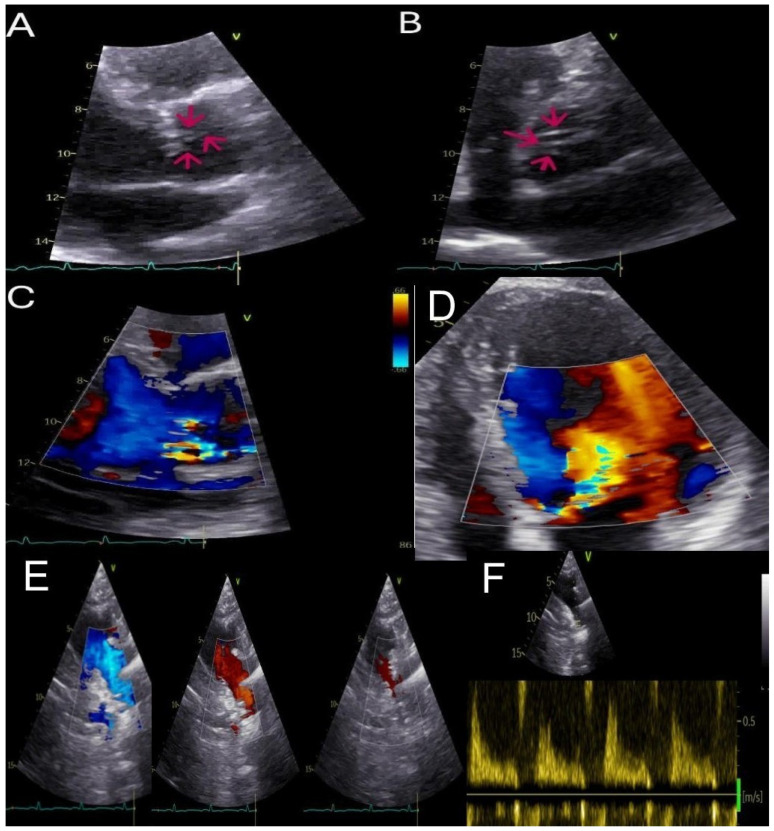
A 49-year-old male patient, overweight with a history of arterial hypertension and moderate aortic regurgitation with mild symptoms, was referred to our department for appropriate management. Trans-thoracic echocardiography (TTE) revealed moderate-to-severe aortic regurgitation following a multiparametric evaluation [1]: the aortic root (37 mm), annulus (26 mm), and sinotubular junction (30 mm) were of normal size, and the aortic valve was tricuspid, with normal systolic movement of the leaflets. During diastolic closure, subtle hyperechoic filaments of uncertain characterization were noted as shown by red arrows (PLAX 2D, panel (A); PSAX 2D, panel (B); Supplementary Video S1). Doppler evaluation demonstrated an eccentric regurgitant jet directed posteriorly, with a vena contracta (VC) of 5 mm (see, PLAX 2D color, panel (C)), which led to anterior mitral leaflet (AML) doming (see, apical 4CH view 2D color showing color aliasing of the aortic regurgitation jet along the entire anterior mitral leaflet, panel (D)). Although it was not possible to reliably measure the pressure half-time (PHT), other indicators of hemodynamic significance were identified. These included retrograde holodiastolic flow in the descending aorta with a peak end-diastolic reverse velocity of 0.26 m/s, as shown by color Doppler imaging (suprasternal 2D color view, from left to right proto-diastolic, mid-diastolic, tele-diastolic timeframes, panel (E); suprasternal 2D view with sample volume in the descending aorta, panel (F)), in addition to an increase in left ventricular end-diastolic volume despite preserved systolic function (end-diastolic volume indexed at 80 mL/m^2^, cardiac output 7.8 L/min, LVEF 55%).
